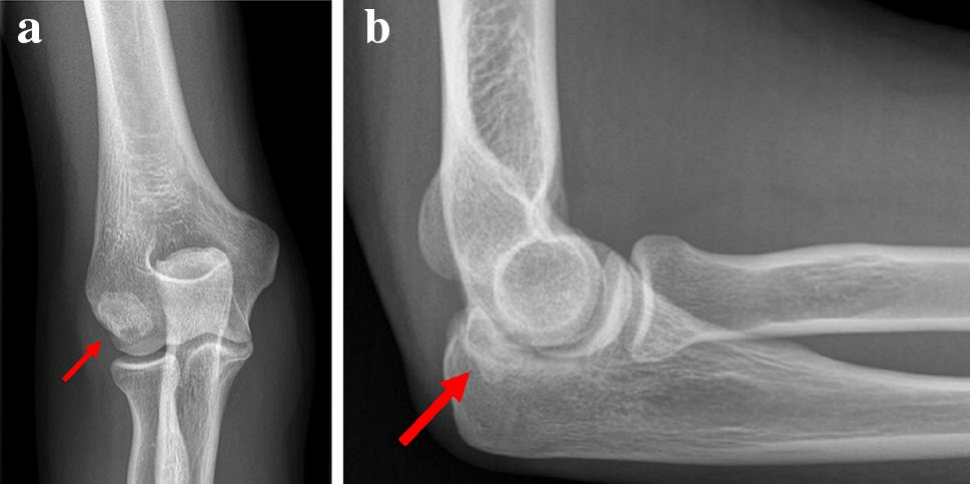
a Anteroposterior radiograph of the right elbow of patient no. 10 at first presentation (the arrow marks the new bone formation). b Lateral radiograph of the right elbow of patient no. 10 at first presentation (the arrow marks the ossification)
